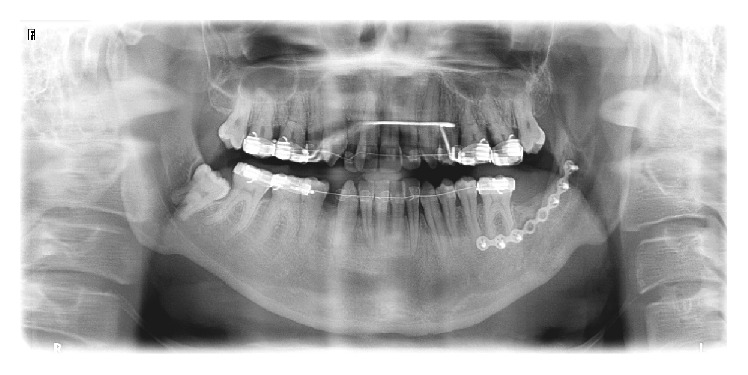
Postoperative control radiography after 6 months of healing period.
